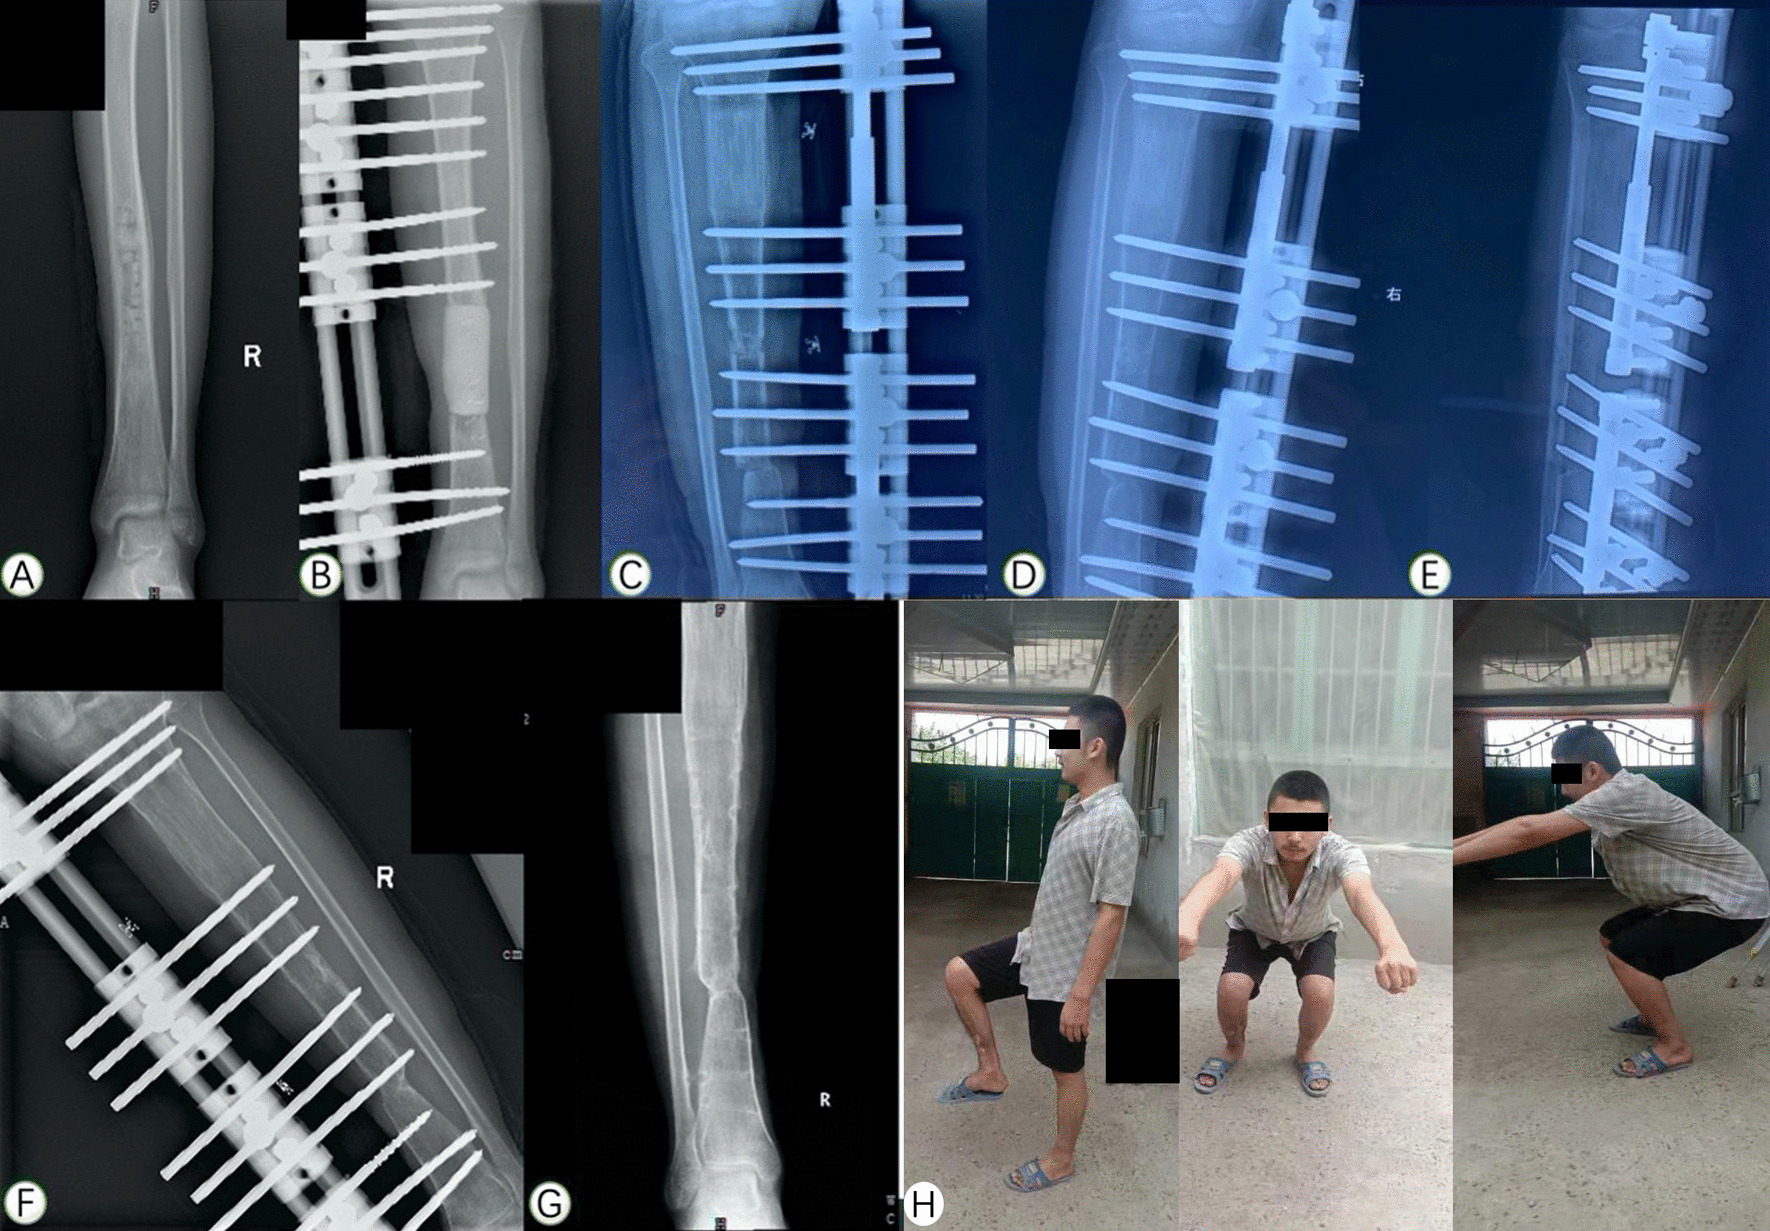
A An 43-year-old male patient with posttraumatic osteomyelitis of the right tibia. B–E After three previous debridement operations, there was a defect of 8.0 cm and filled with PMMA spacer. The soft tissue defect was covered by latissimus dorsi flap. F Two months after bifocal bone transport using monolateral external fixator. G, H External fixator was removed with excellent bone result assessed by ASAMI system 3 years after removal of external fixator. I–K Radiograph and general appearance at last visit with excellent functional result
